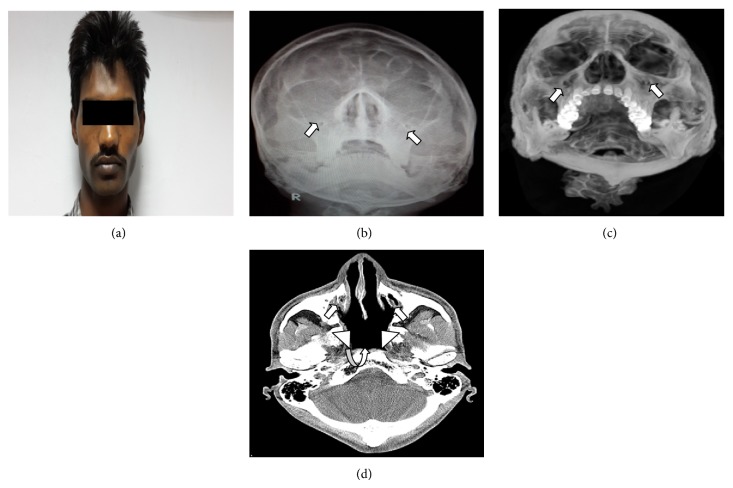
25-year-old male with recurrent headache. Face profile of patient (a). Opacification of bilateral maxillary sinuses (arrow) on plain radiograph (b) with loss of pneumatisation (arrow) on 3D CT reconstruction paranasal sinuses (c). Cleft like bilateral maxillary sinuses (arrow) and absent uncinate process (arrowhead) and enlargement of nasal fossa (curved arrow) on axial section plain CT paranasal sinuses (d).
